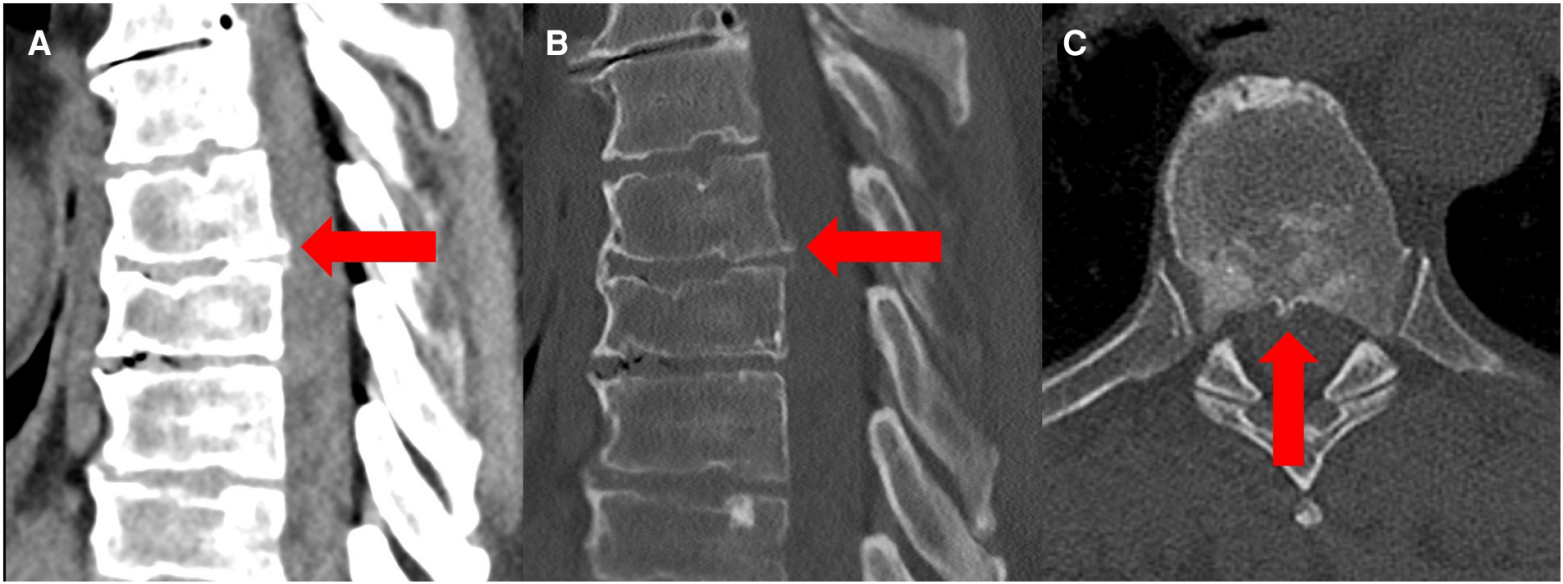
Preoperative sagittal and axial CT scan in a soft tissue window (A) and bone window (B,C) revealed a bone spur at the level T6/7 (red arrow) as a possible cause of spinal cord herniation.
